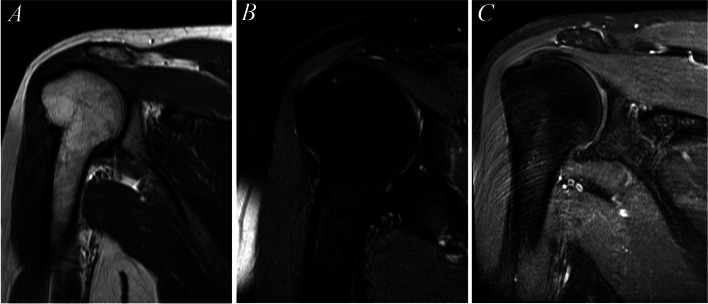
Follow-up MRI (A) resolving of proximal humeral intramedullary signal abnormality and appears organized in appearance with no abnormal high signal intensity in T2 (B) or abnormal enhancement (C). The picture is in keeping with resolving of the osteomyelitis with granulation tissue. No new changes or areas worrisome for infection
